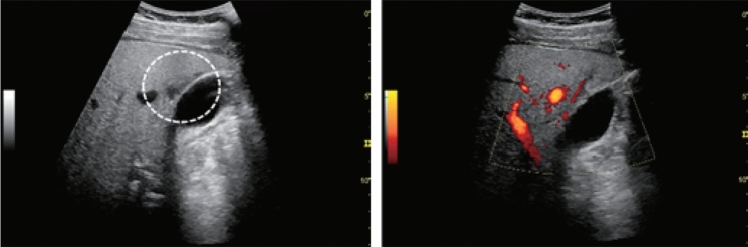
Diffuse lesion. Commonly focal spared area in fatty liver (Around the gallbladder: Cystic vein reflux region). Left: B-mode, Right: Power Doppler. Category 2, Assessment C
